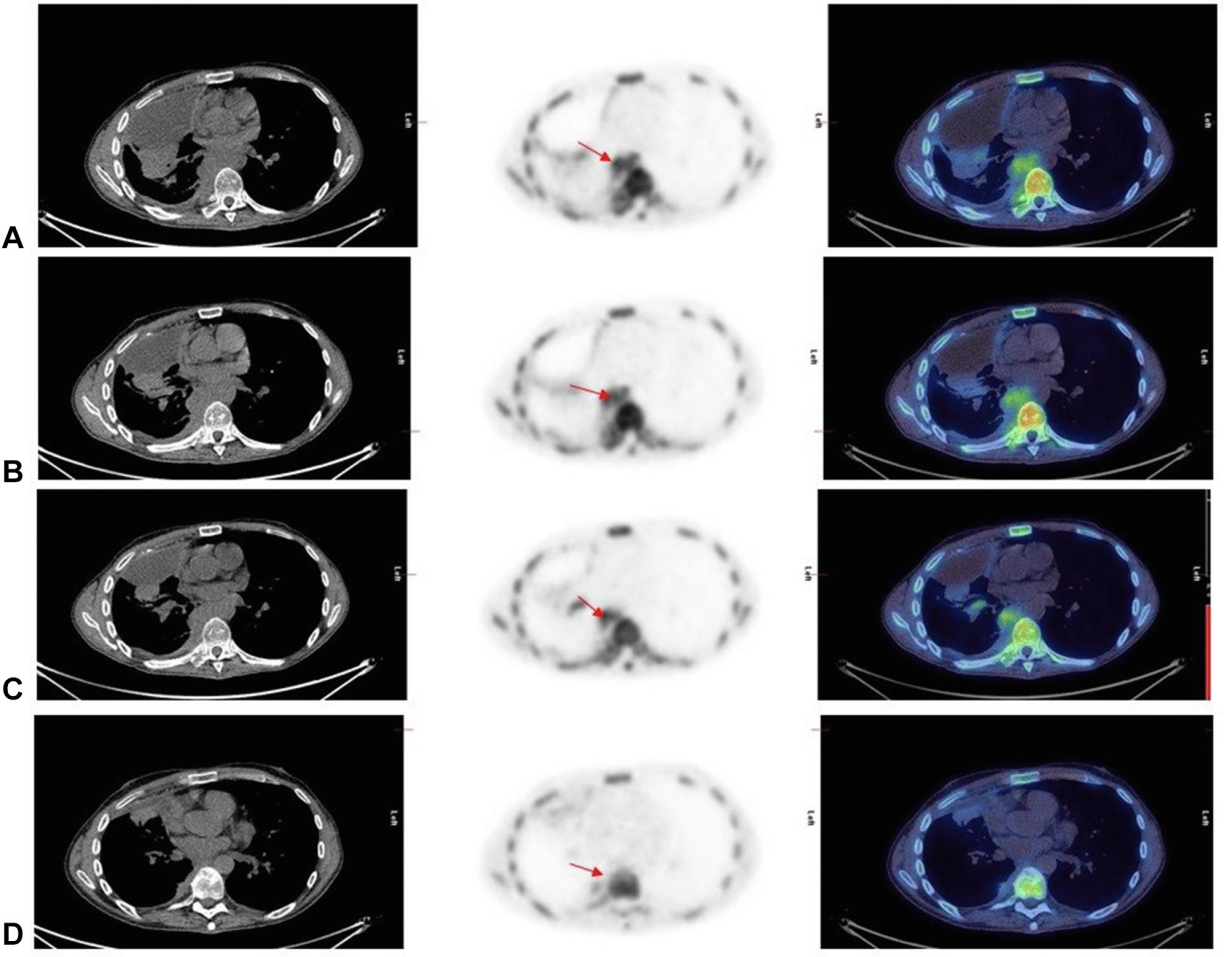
FLT PET-CT in MPM (case 4). (*A*) Baseline SUVmax equals 6.4 (red arrow). (*B*) Post ADI-PEG20 at 24 hours, the SUVmax decreased to 5.9 (8% reduction hence StD). (*C*) Postcycle 1 of combined therapy, the SUVmax increased slightly to 6.2 (maintained StD); however, at (*D*) end of treatment, the SUVmax decreased significantly to 1.0 (84% reduction from baseline hence PR). CT also had response, but StD at EOT and earlier time points. ADI-PEG20, pegargiminase; CT, computed tomography; EOT, end of treatment; FLT, 3'-deoxy-3'-fluorothymidine; MPM, malignant pleural mesothelioma; PET, positron emission tomography; PR, partial response; StD, stable disease; SUVmax, maximum standardized uptake value.
